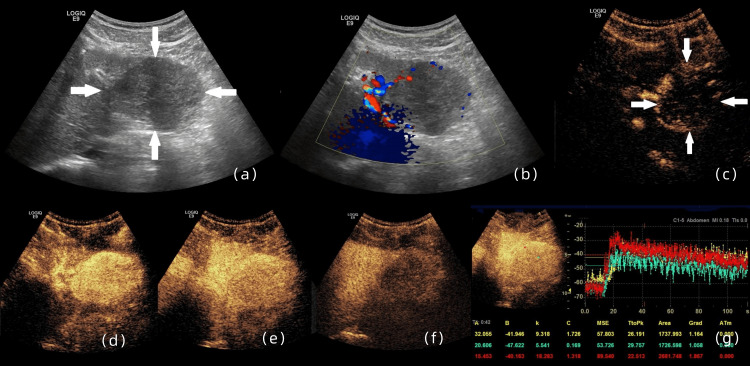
Ultrasonographic characterization of the hepatic lesion (a) B-mode ultrasonography demonstrates a well-demarcated hypoechoic mass (6.5 × 4.8 cm) with ovoid morphology in the left hepatic lobe. (b) Color Doppler imaging identifies circumferential vascularity along the lesion periphery. (c) Early arterial phase (13 seconds postcontrast) exhibits annular hyperenhancement preceding adjacent parenchymal opacification. (d) Complete centripetal filling with homogeneous hyperenhancement is achieved by 19 seconds. (e) Portal phase imaging reveals mild central hypoenhancement, contrasting with sustained peripheral hyperenhancement. (f) Delayed phase (four minutes 25 seconds) demonstrates near-complete contrast washout. (g) Time-intensity curve analysis (ROI annotations: red, perilesional zone; green, central lesion; yellow, adjacent parenchyma) delineates three-phase enhancement kinetics: The enhancement of the lesion preceded that of the adjacent liver parenchyma, with the highest level of enhancement seen at its periphery and the earliest clearance observed in its central part. The area under the time-intensity curve indicated that the lesion periphery had a higher intensity than the adjacent liver parenchyma, which in turn, had a higher level of intensity than the lesional interior
